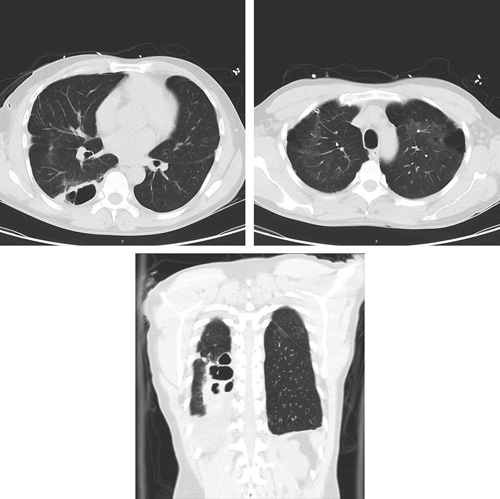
(High resolution CT Scan of chest) Diffuse emphysematous changes, bullous changes of the lateral aspect of both upper lobes, greater on the left, nonspecific mild ground glass infiltrates in the posterior aspect of the right lower lobe with adjacent bullous changes but resolution of pneumothorax. CT, computed tomography.
